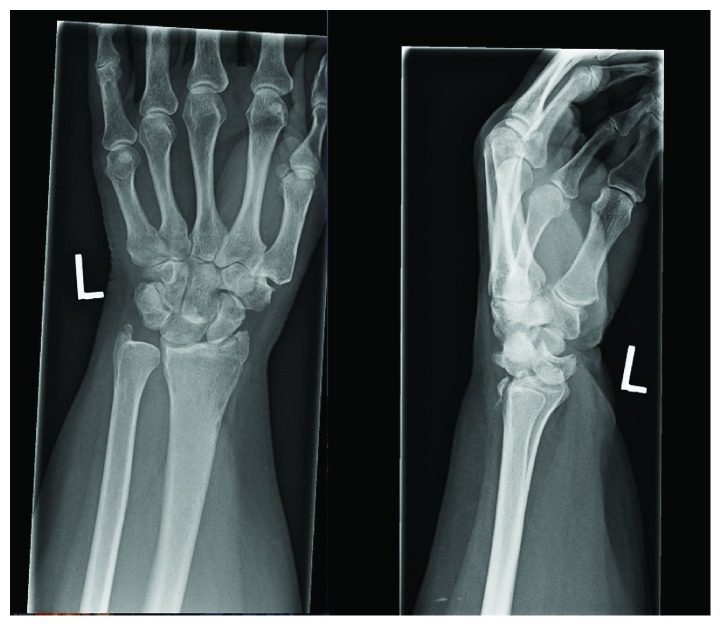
AP and lateral images of the initial radiographs taken in the peripheral hospital. Note the comminuted scaphoid facet, subtle triquetral fracture (AP) as well as the obvious perilunate dislocation (lateral).
